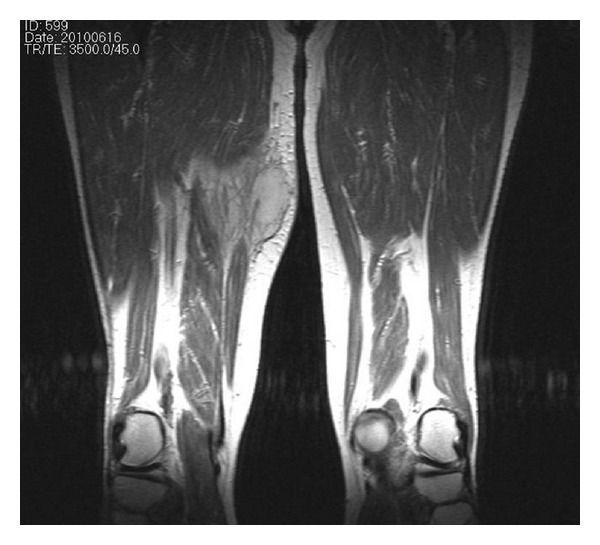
T2-weighted sagittal MRI of the thigh showing a heterogenous mass in the medial aspect of the right thigh.
